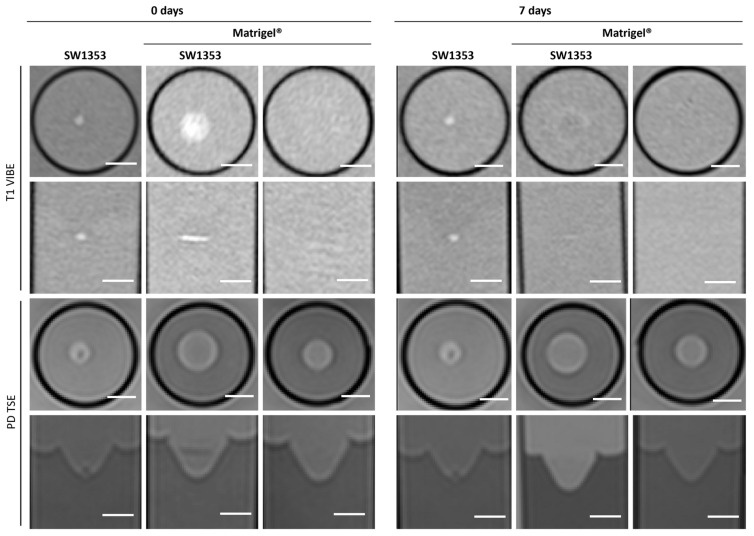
Cross-sectional (1st and 3rd row) and sagittal (2nd and 4th row) images of SW1353 spheroids without and with Matrigel^®^ and just Matrigel^®^. T1-weighted images (1st and 2nd row were obtained using a spoiled gradient echo VIBE sequence. PD-weighted images were obtained using a TSE sequence. Scale bars represent 10 mm.
